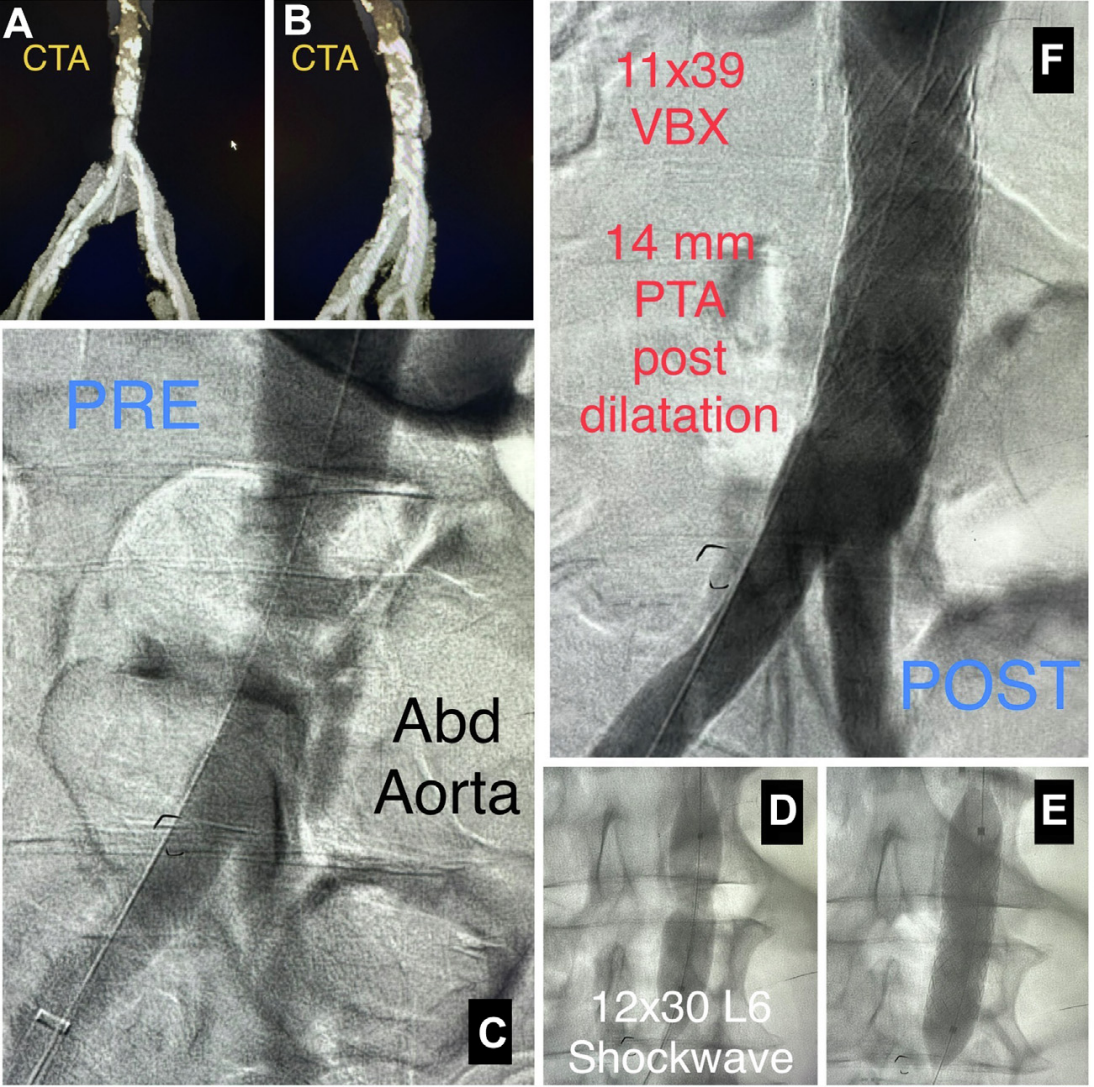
Abdominal aorta procedural components and imaging. (A, B) Computed tomography angiography (CTA) of abdominal aorta and iliac arteries. (C) Diagnostic angiogram abdominal aorta. (D) Intravascular lithotripsy (IVL) of abdominal aorta using a 12.0- × 30.0-mm Shockwave L6 balloon. (E) Deployment of 11.0- × 39.0-mm Viabahn VBX balloon expandable covered stent. (F) Final angiogram of abdominal aorta after IVL and stent deployment.
